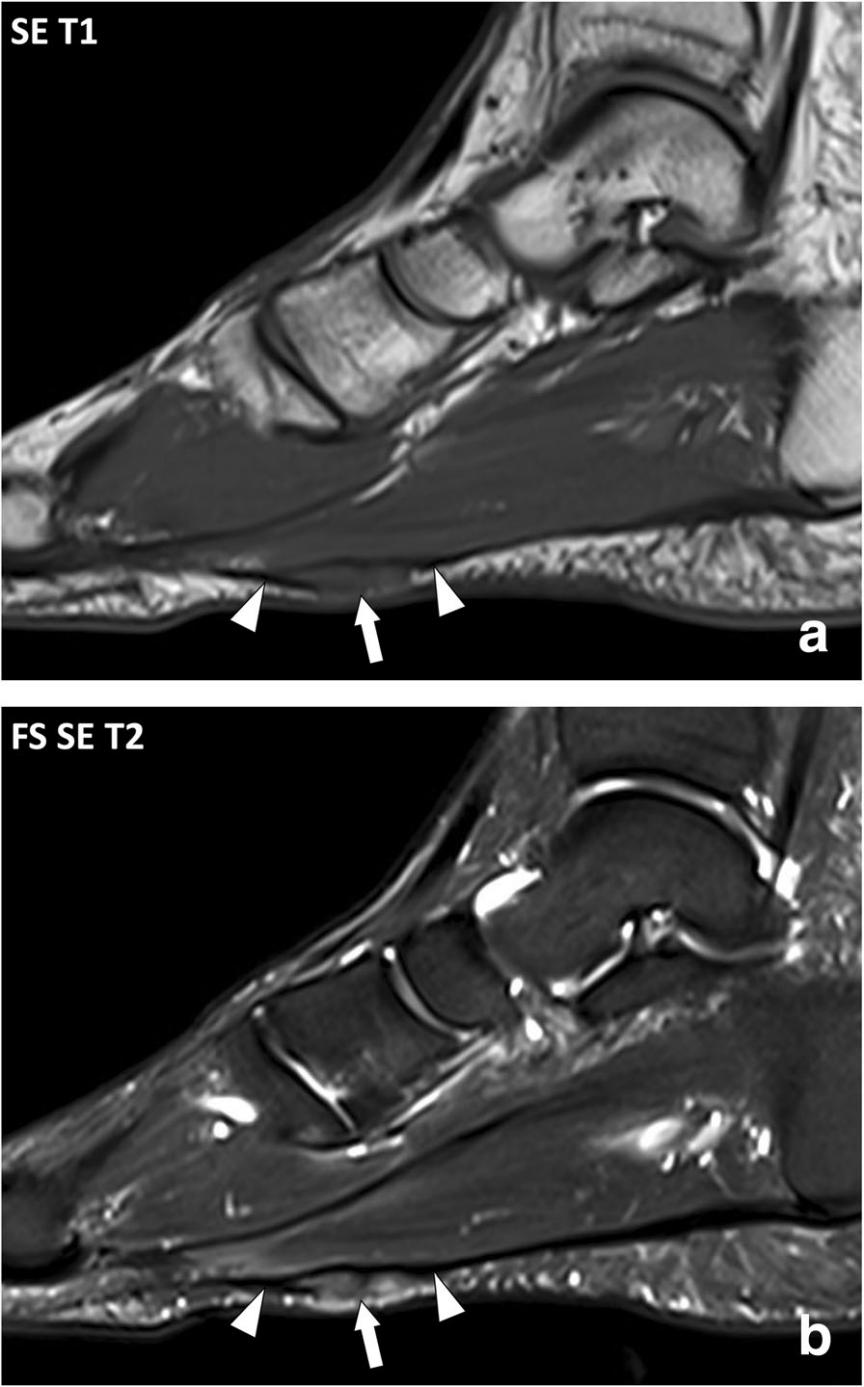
Sagittal (a) SE T1w and (b) fat-suppressed SE T2w images of the right foot of a 47-year-old male with plantar fibromatosis. MRI demonstrates fusiform thickening of the plantar fibromatosis with a nodule of low signal intensity on the T1w image and heterogeneous high signal intensity on the T2w image (arrow) in continuity with the normal aponeurosis (arrowheads)
